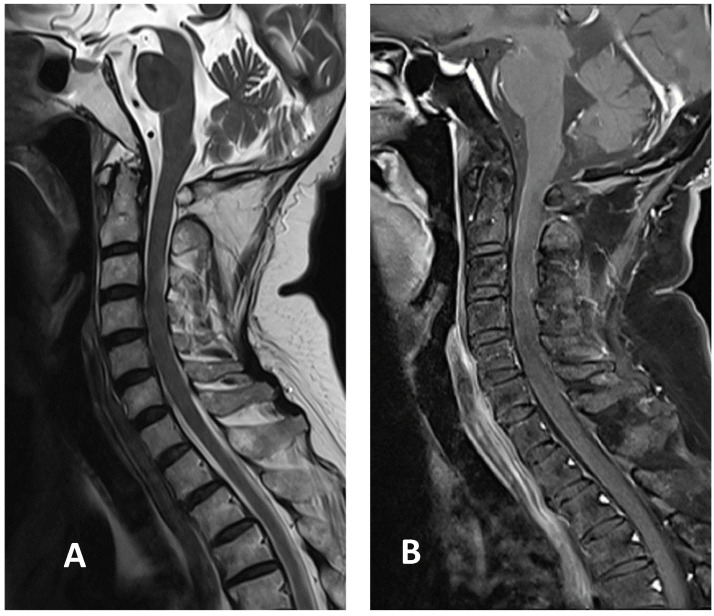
Cervical MRI images two weeks after discharge: (A) sagittal T2-weighted image showing the decreased size of hyperintensity in the cervical cord at the C3 level; (B) sagittal T1-weighted image with contrast showing minimal enhancement in the cervical cord at the C3 level.
